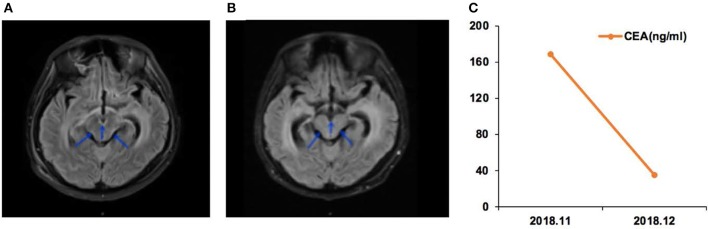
Case 01 was a male of 34 years of age, with lung adenocarcinoma and with a history of smoking, but quitted 10 years ago. (A) T2 FLAIR enhanced magnetic resonance imaging (MRI) showed abnormal high signal in the medulla, oblongata, pon, and ventral and dorsal midbrain, suggesting leptomeningeal metastases (LMs). (B) T2 FLAIR enhanced MRI during afatinib treatment showed that the abnormal high signal in the medulla, oblongata, and ventral and dorsal midbrain was lower than before treatment. (C) Carcinoembryonic antigen (CEA) levels before and after afatinib treatment.
